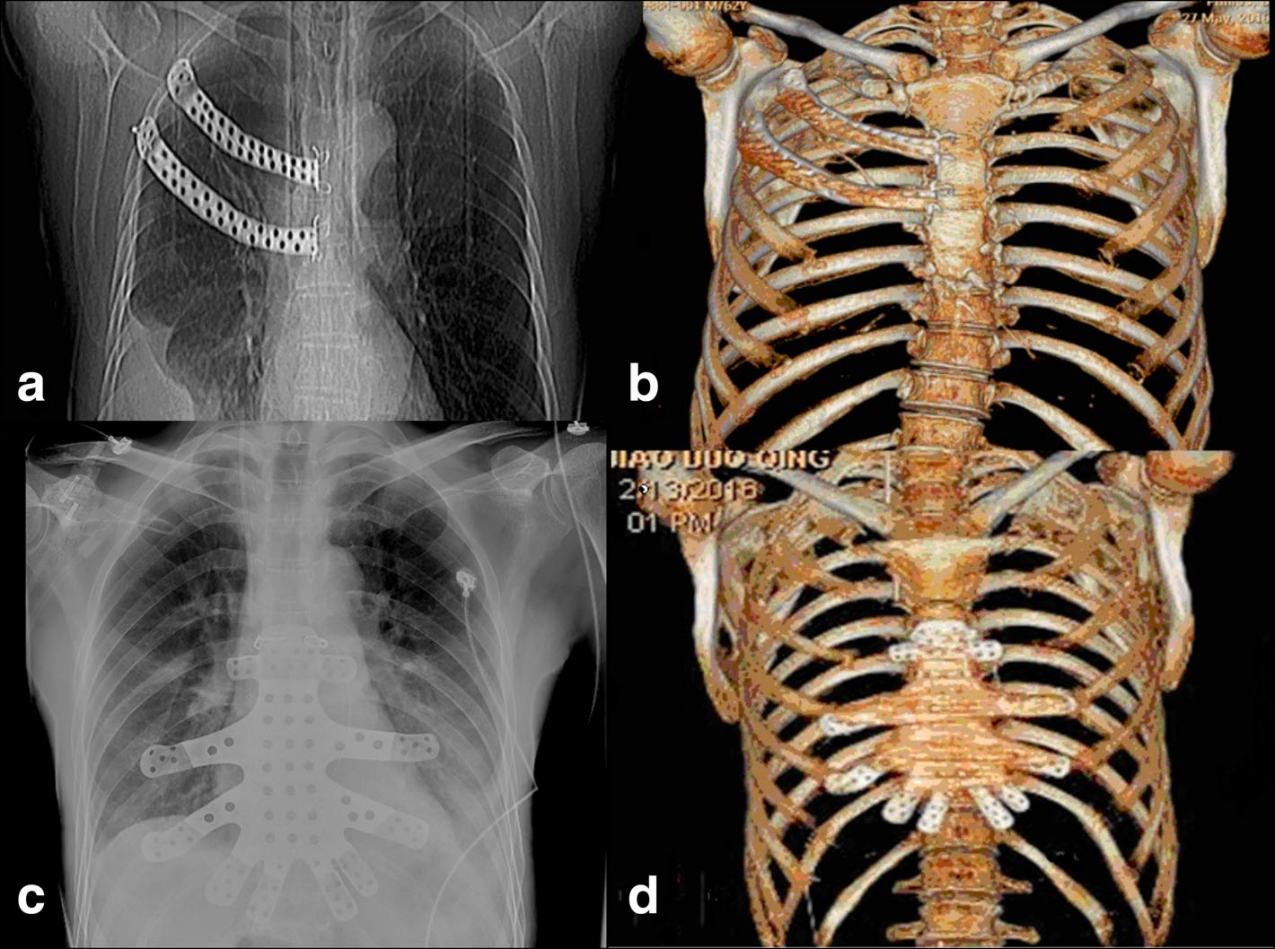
a, c Postoperative X-ray images showing that the prostheses fit well in the chest. b, d 3D reconstructions of CT data showing that the prostheses were well fixed in the chest
